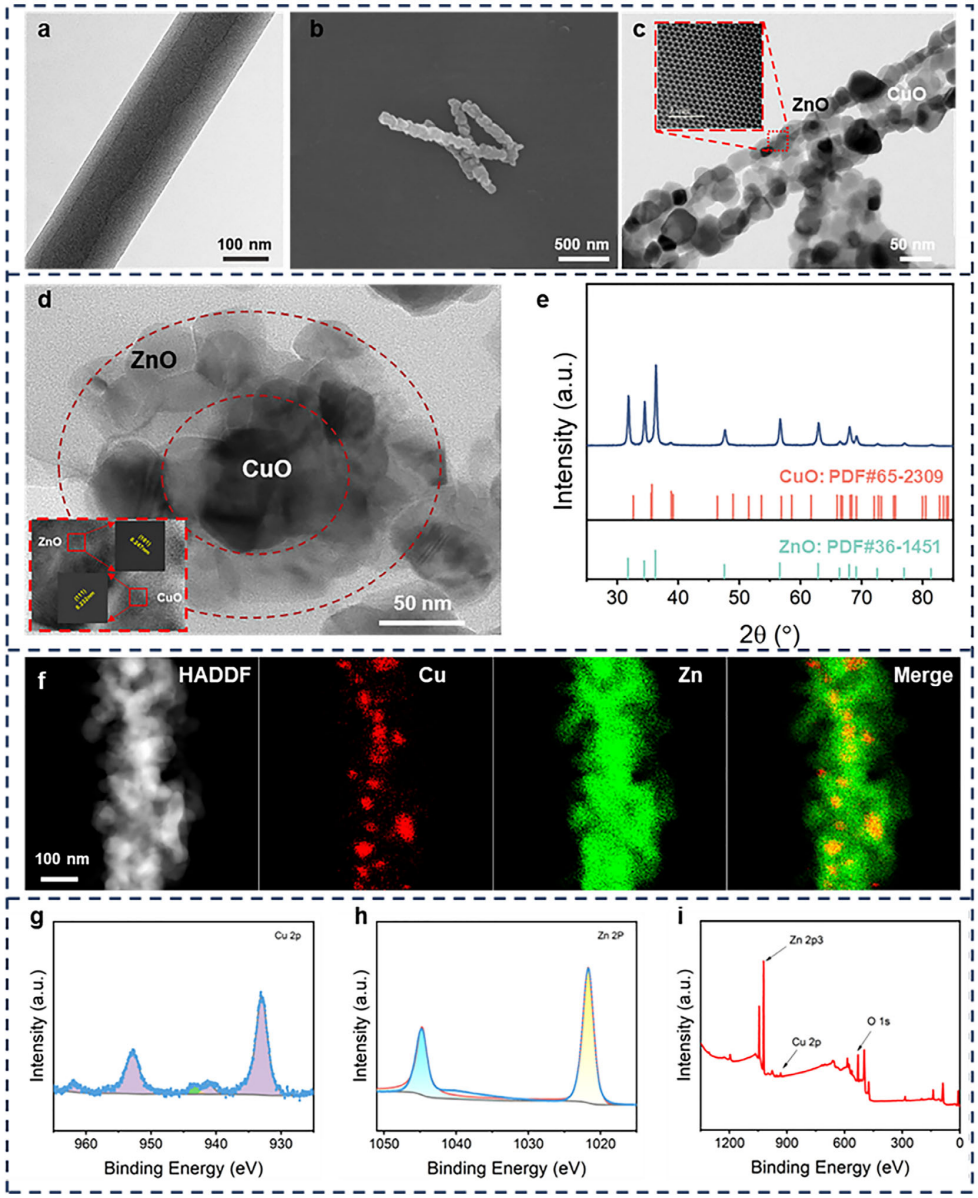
Characterization of the coaxial structure of ZnO@CuO nanofibers with inner CuO layer and outer ZnO layer. (a) TEM image of ZnO@CuO coaxial nanofiber precursor fibers. (b) SEM image of ZnO@CuO coaxial nanofibers after ultrasonic dispersion. HRTEM images of axial (c) and cross‐sectional (d) surfaces of ZnO@CuO coaxial nanofibers. (e) XRD pattern, (f) EDS mapping by AC‐TEM, and (g‐i) XPS patterns of ZnO@CuO coaxial nanofibers.
